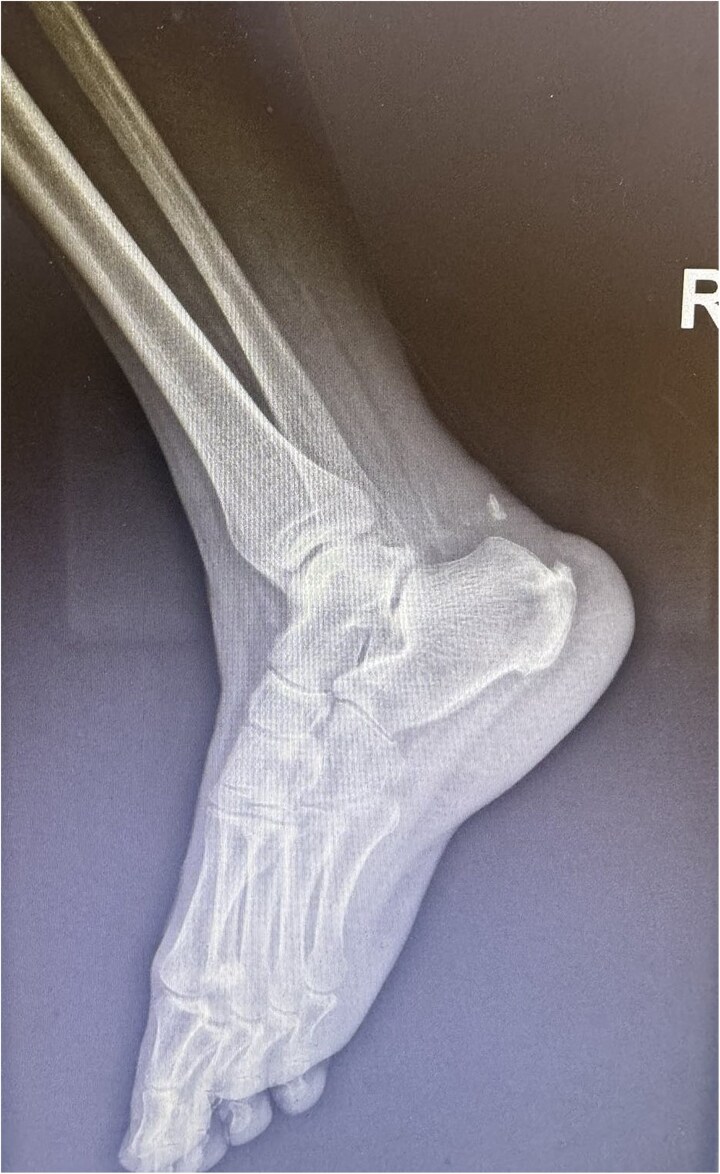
X-ray of Achilles tendon rupture with bone avulsion.
